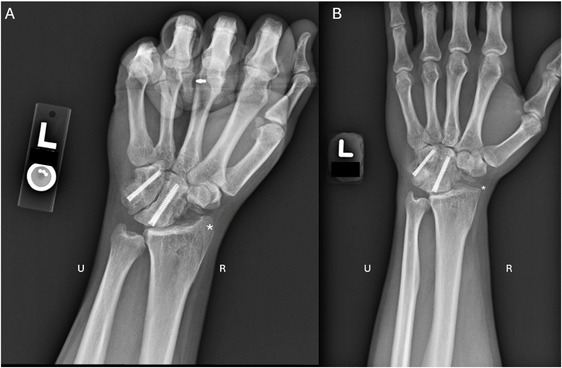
Left, supine on hand table in wrist traction tower. (A) Preoperative radiograph with prominent radial styloid (*). (B) 8‐week postoperative radiograph status post radial styloid resection (*). (R, radial; U, ulnar.)
